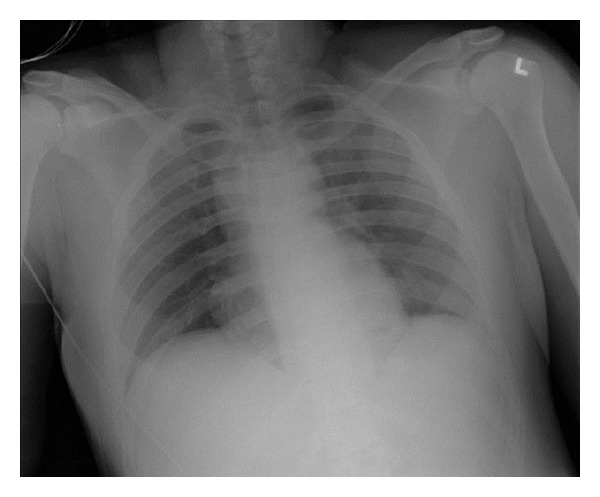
An AP chest X-ray in the trauma bay showed no obvious pneumothorax, hemothorax, or bullet fragment.
